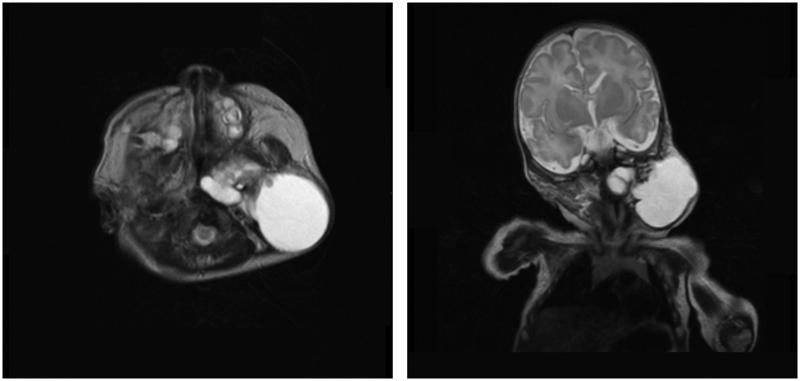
T2 weighted MRI at 1-month-old. The mass was multicystic and located near pharynx and parotid gland.
